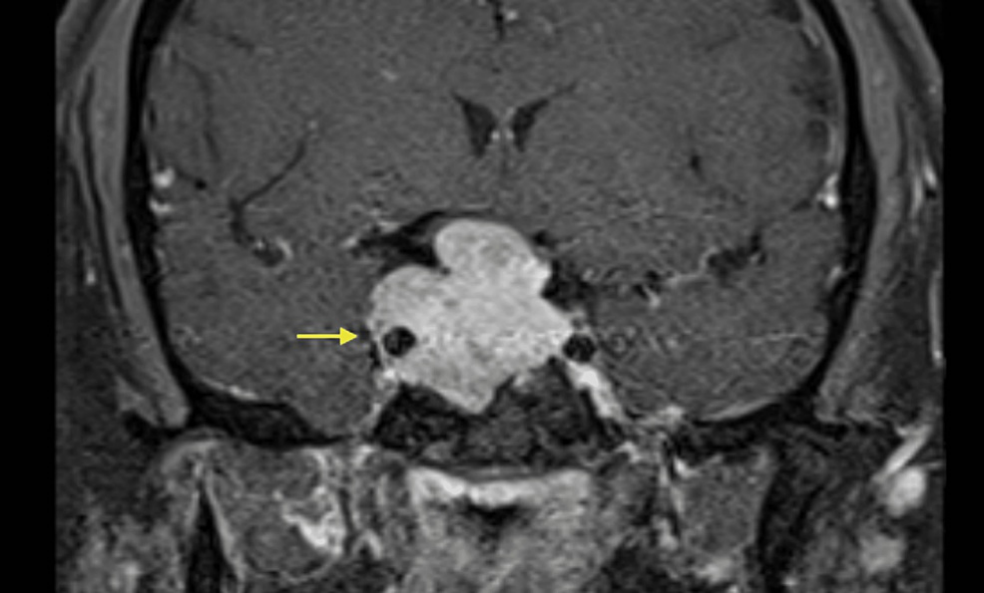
Pre-operative coronal MRI of pituitary mass T1 post-contrast fat saturation showing contrast enhancement of a large sellar/suprasellar mass with encasement of the cavernous portion of the right internal carotid artery (yellow arrow).
